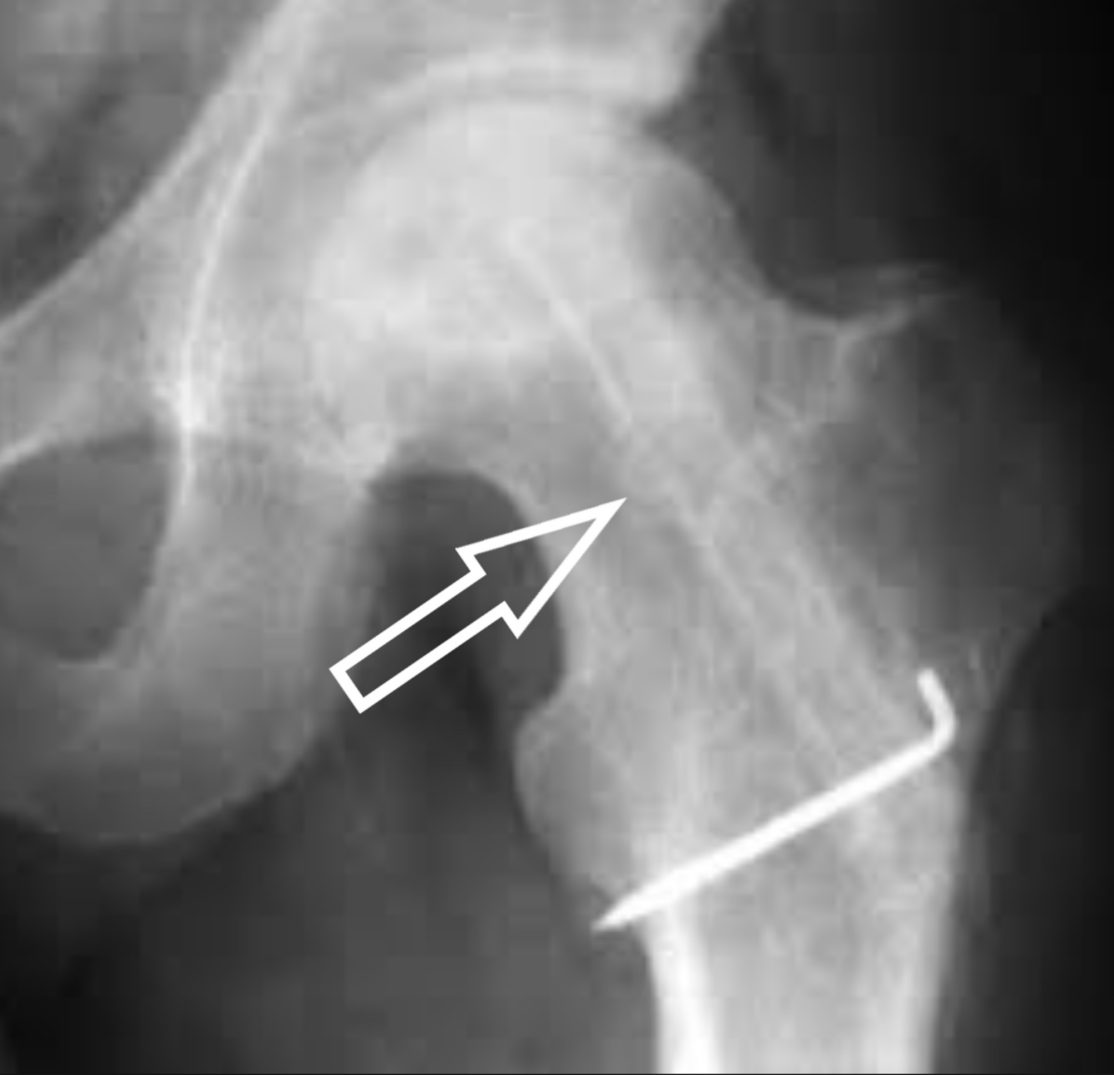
Post-op grafting X-ray X-ray showing bone grafting after decompression with the help of k-wires (Kirschner wires).
